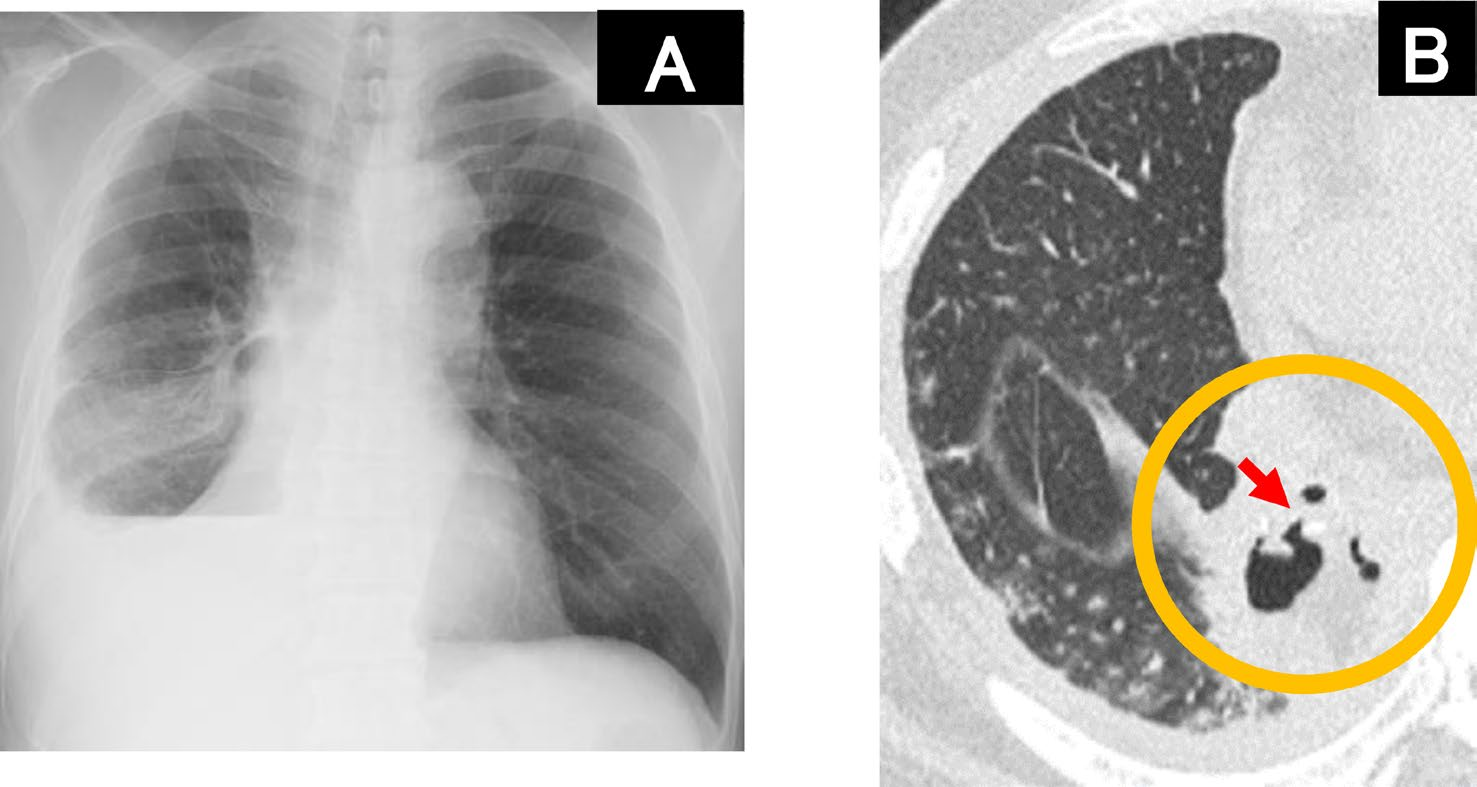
Radiological images on Day 35. (A) Chest radiograph shows a worsening cystic shadow with niveau in the right lower lobe. (B) Chest computed tomography showing an infiltrating shadow and fistula at the suture of the right lower lobe.
